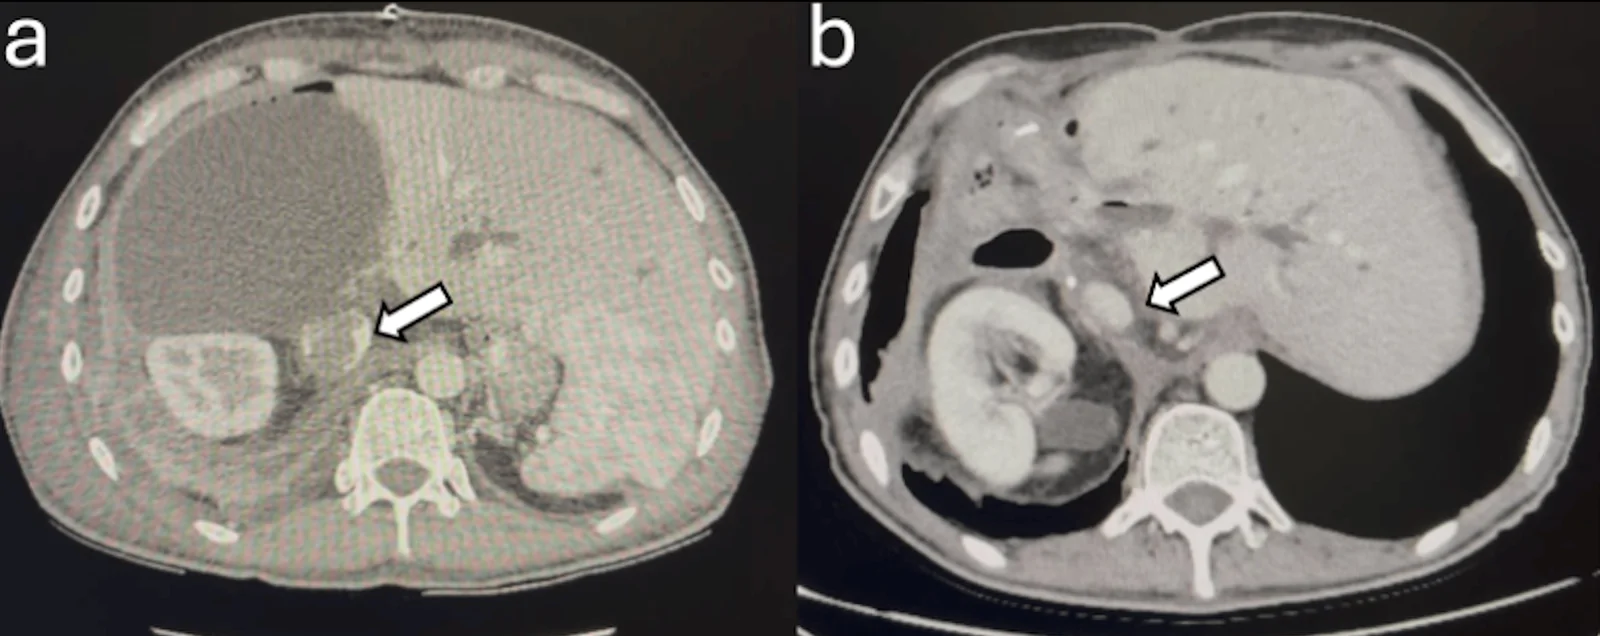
Contrast-enhanced abdominal CT findings.
